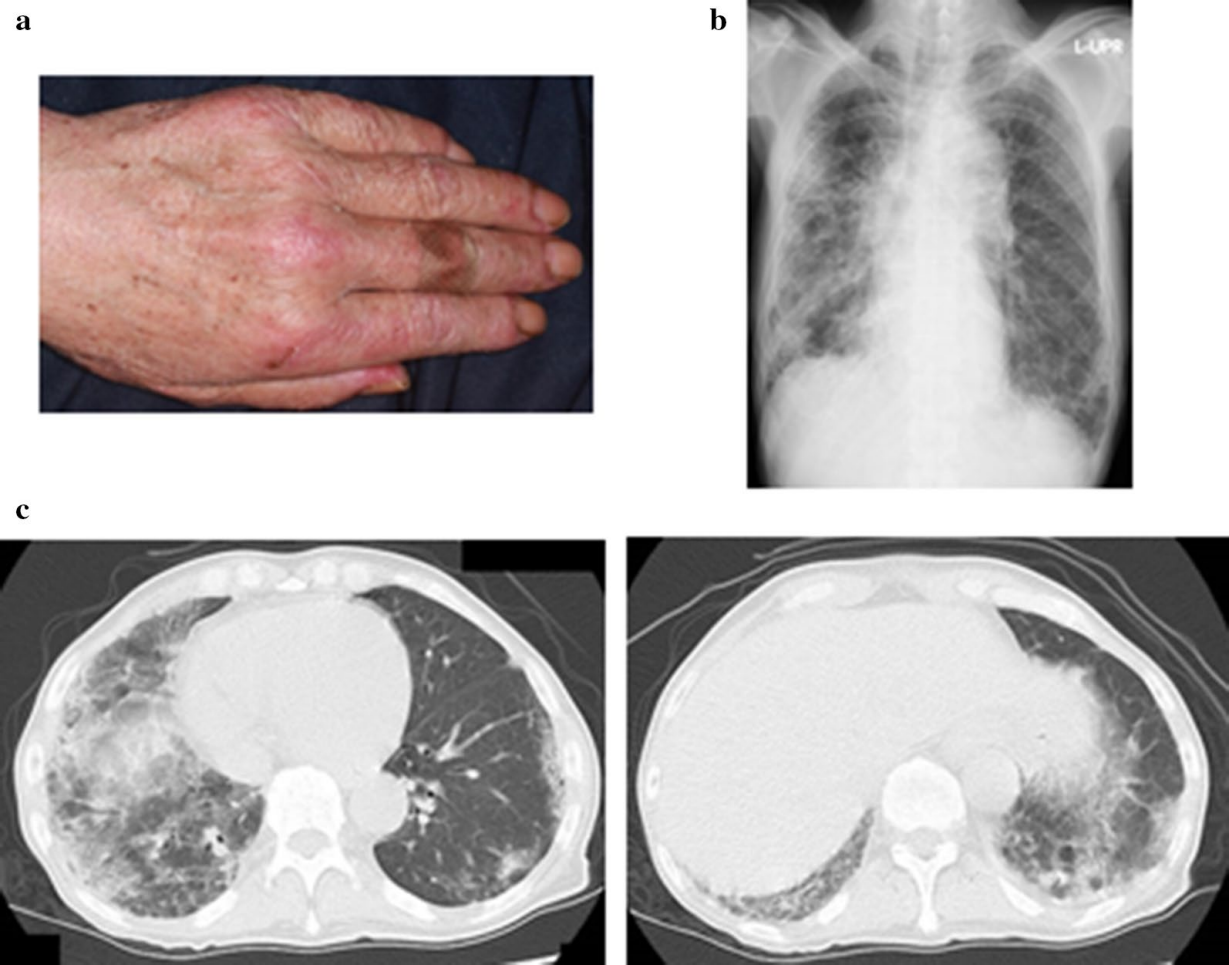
a The clinical presentation on admission. Papules were observed near the interphalangeal articulations (Gottron’s sign). b Chest radiography showed bilateral reticular shadows, in the right lung. c Chest CT scan showed bilateral, asymmetric, ground-glass opacities and reticular shadows, predominantly in the lower lung
